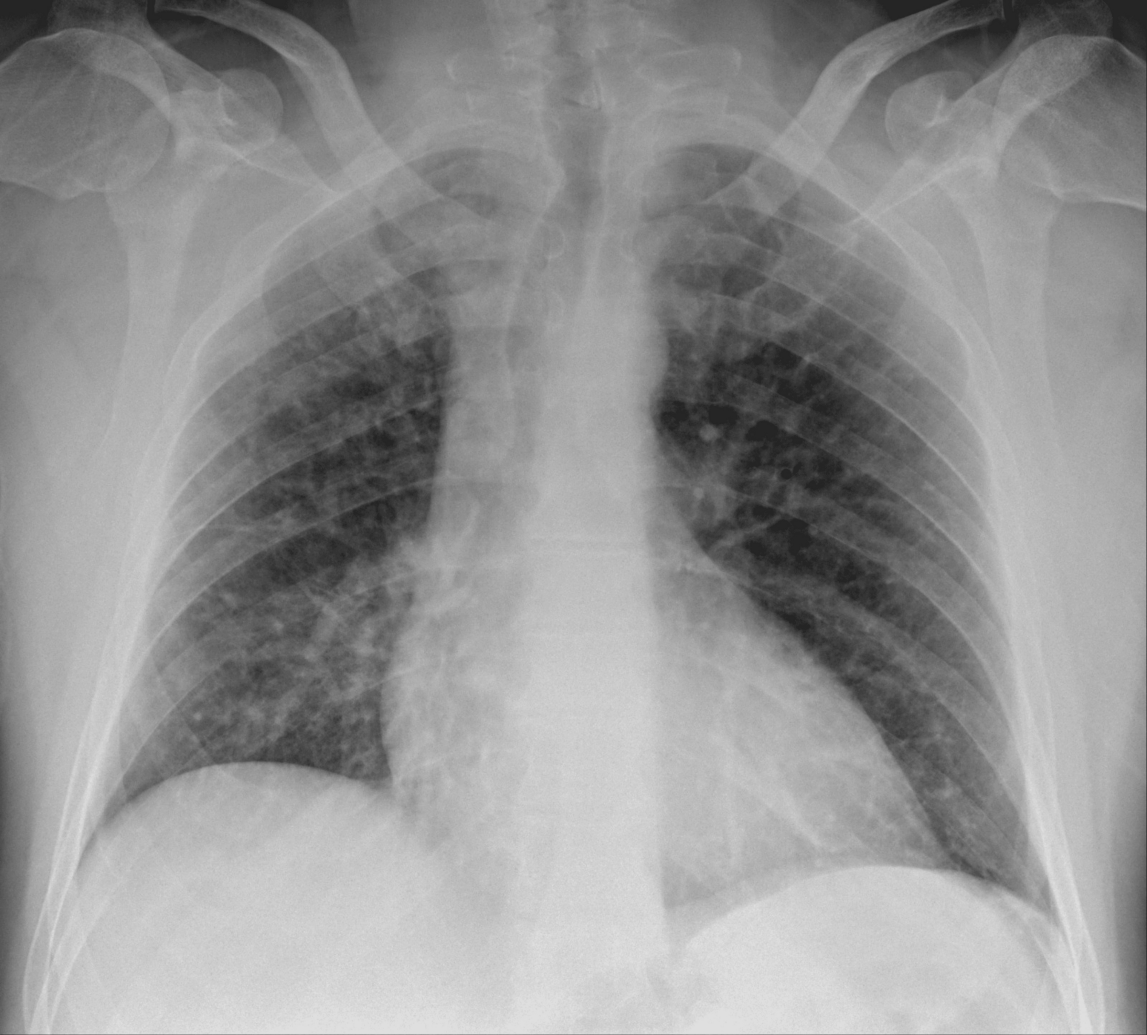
Diffuse reticulonodular infiltrates in the chest X-ray
